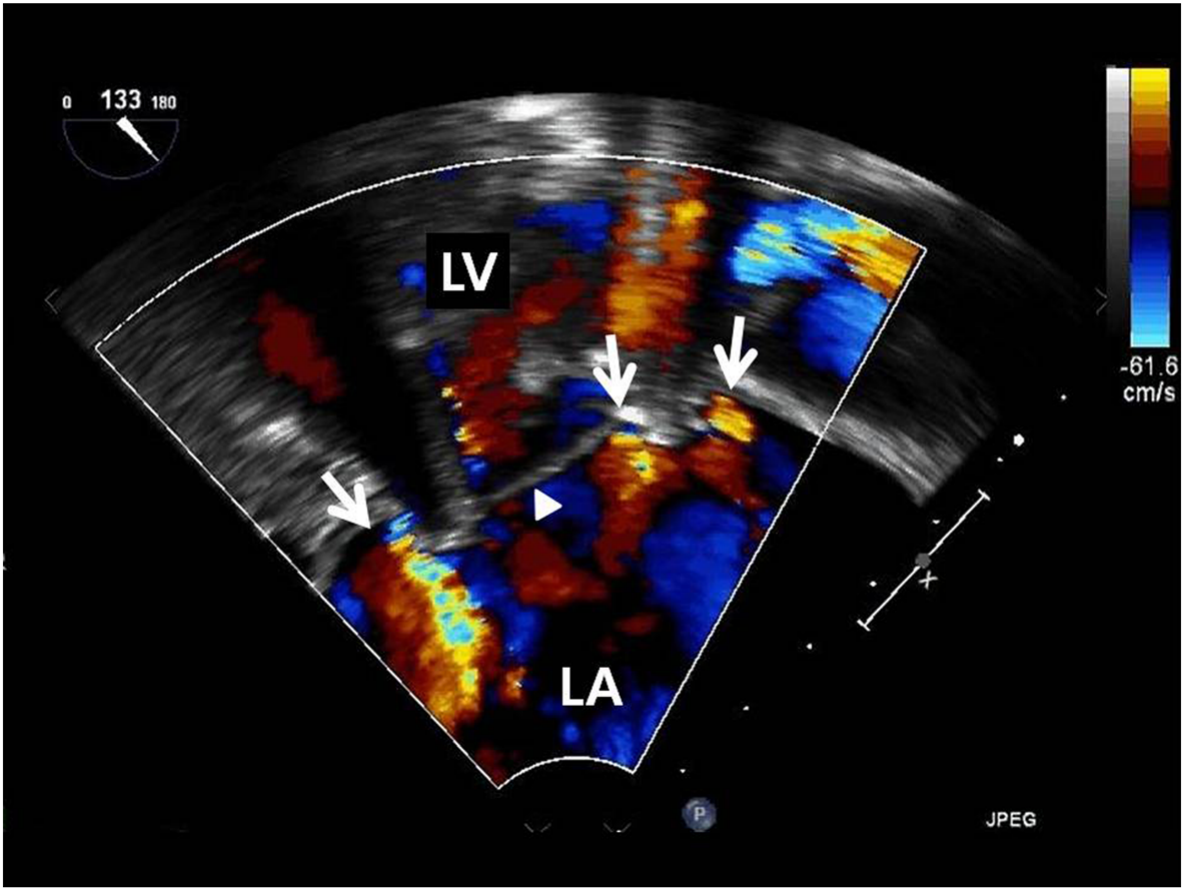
Preoperative echocardiographic finding shows multiple paravalvular leaks in the mitral valve. The arrow indicates multiple mitral regurgitant flow and the arrow head indicates the mechanical mitral valve (LA, left atrium; LV, left ventricle).
